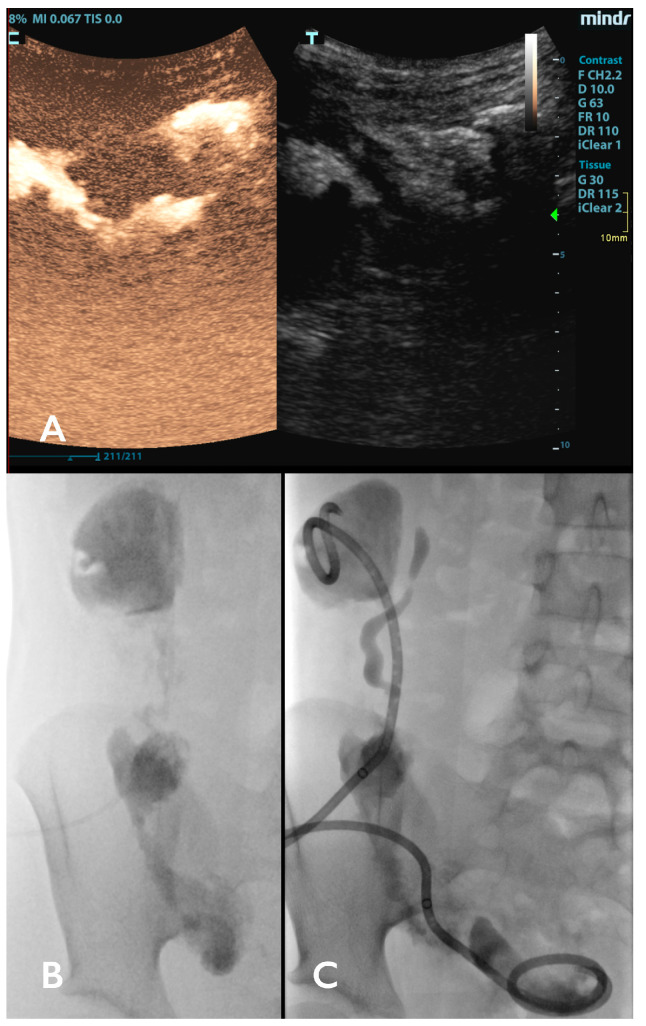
(A) One week after the IPAD, no clinical improvement was observed in the patient, and a follow-up contrast-enhanced ultrasound was performed injecting the SonoVue^®^ contrast directly into the abscess cavity using the inserted drainage catheter. CEUS revealed the communication between the iliac and paracolic abscess. (B) Another IPAD was immediately performed. The iodine contrast applied during fluoroscopy revealed three communicating abscess cavities that filled with the contrast applied under pressure, but the paracolic cavity then failed to drain upon iliac cavity drainage. (C) Then, a separate paracolic 10F catheter was inserted in addition to another new catheter, placed in the retrovesical abscess cavity. After ten days, the US follow-up revealed no signs of abscess (not depicted), and the patient was discharged.
